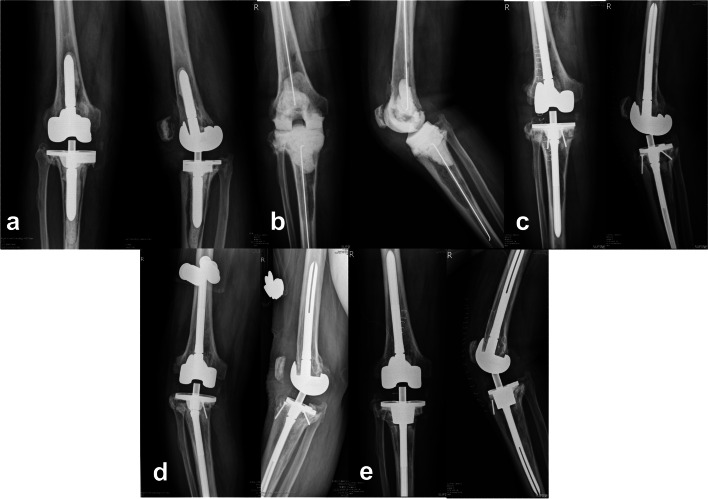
Radiographs of a 78-year-old male showing a) septic status of a left total knee arthroplasty b) status after 1st-stage ALBCS implantation and c) status after 2nd stage revision with reconstruction of structural allograft d) tibia component breakage with allograft resorption 32 months after revision e) status after revision surgery with TM cone reconstruction
